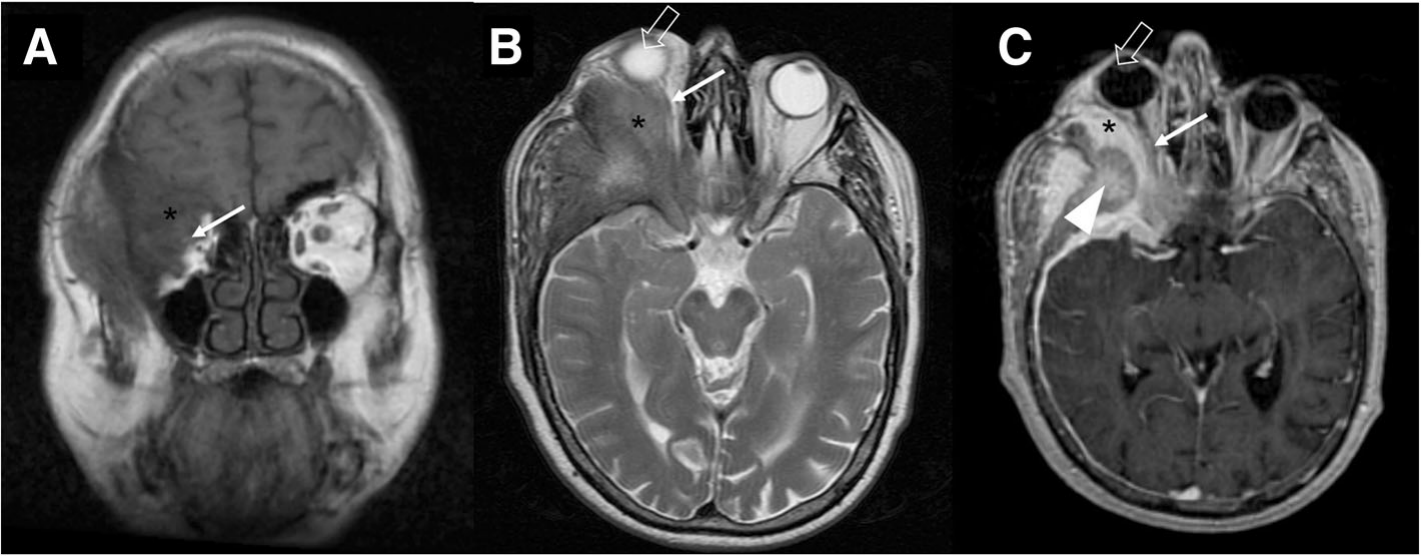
Sphenoid wing meningioma. MRI T1-weighted coronal image (a), T2-weighted (b), and T1-post gadolinium (c) axial images. A large mass with avid enhancement is visible, suggestive for meningioma of the right sphenoid wing (asterisks) with orbital involvement, displacement of the optic nerve (arrows), and the globe (empty arrows). Signs of hyperostosis are also evident (arrowhead)
